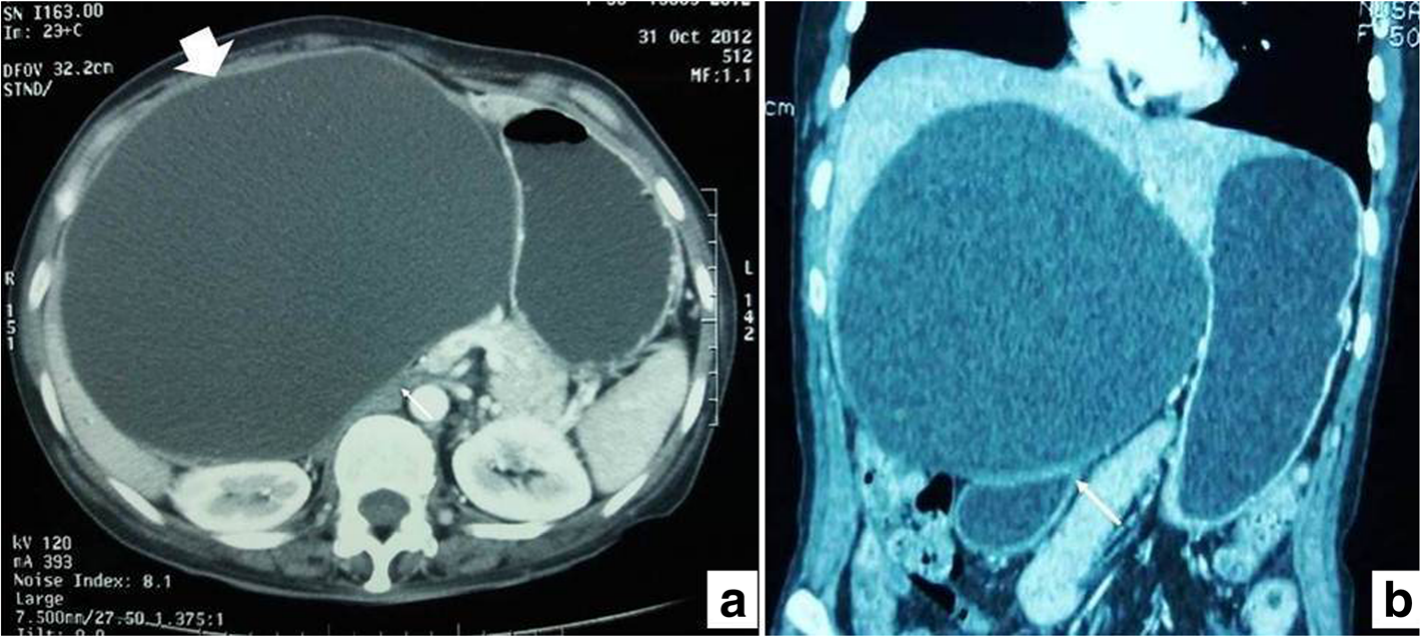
Axial and coronal sections from contrast-enhanced computed tomography of the abdomen. a There is a well-circumscribed, intrahepatic cystic lesion having thin walls (*bold arrow*). There are no internal septations, mural nodules, or abnormal wall enhancement, favoring diagnosis of cystadenoma. b There is significant associated mass effect resulting in compression over duodenum (*thin arrow*)
